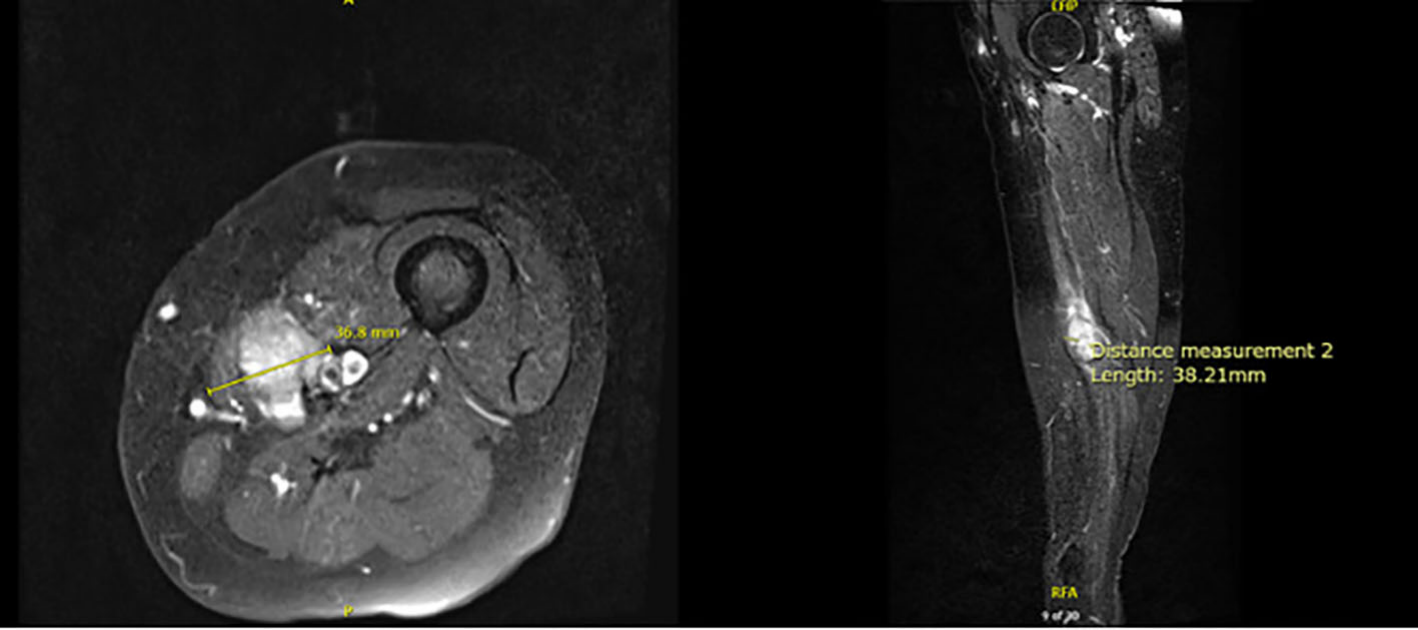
MRI of bilateral lower extremities with contrast in July 2024.
